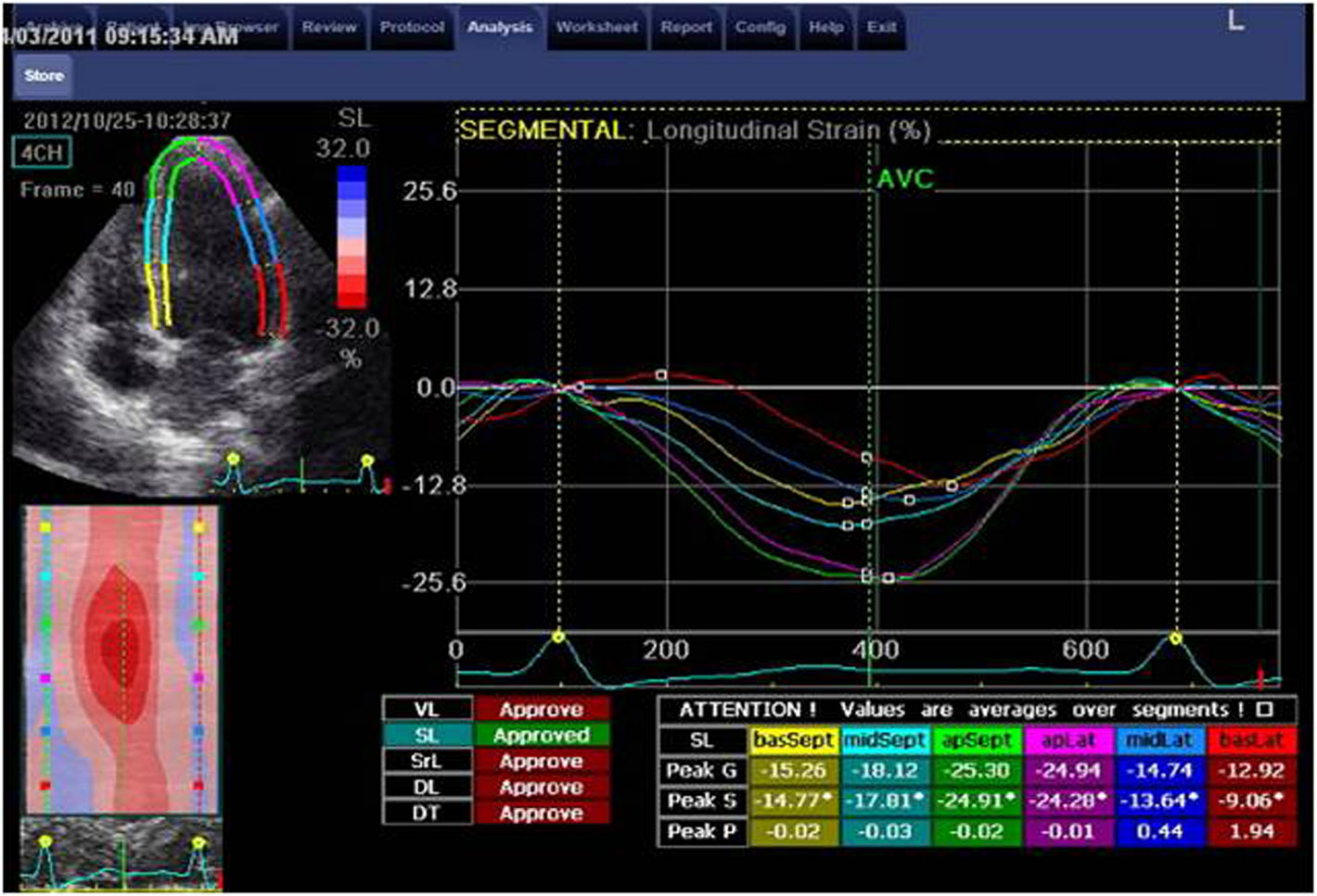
Apical four-chamber view and analysis of septal and lateral wall strain using TTE
